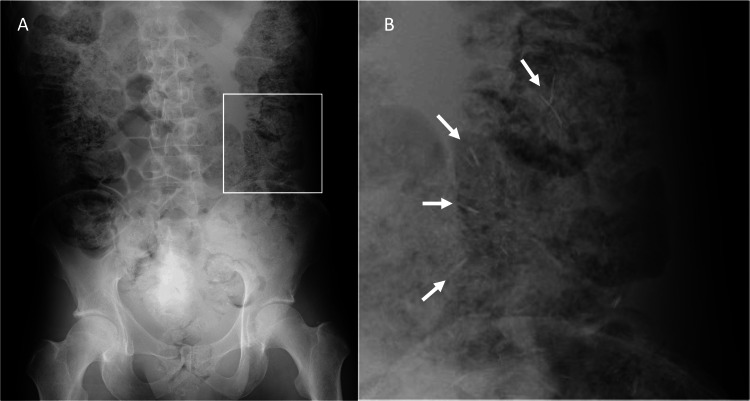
Abdominal X-ray (A) Prominent gas and a large amount of feces in the entire colon. (B) Enlarged view of the white square area of (A). A large amount of thin fibrous materials present within the feces.
